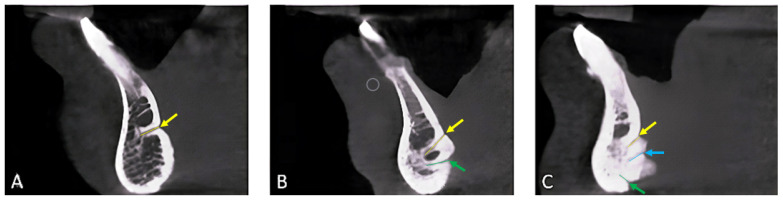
Sagittal view at the mandibular lingual canal/s of CBCT scan showing three different variations, (A) single, (B) double, (C) triple canals. The yellow arrow points to the supra-spinosum foramen, which is the opening of its corresponding canal (i.e., yellow line). The blue arrow points to the inter-spinosum foramen, which is the opening of its corresponding canal (i.e., blue line). The green arrow points to the infra-spinosum foramen, which is the opening of its corresponding canal (i.e., green line).
